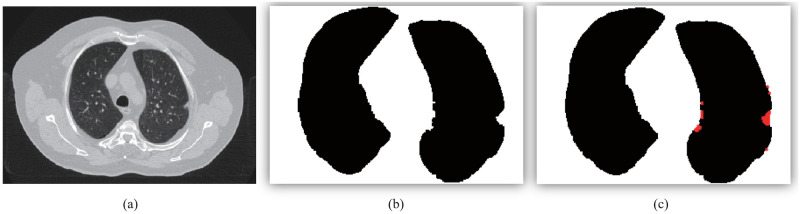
The correction effect comparison chart. (a) The original CT slice; (b) the extracted coarse lung binary region; (c) the corrected lung region.
